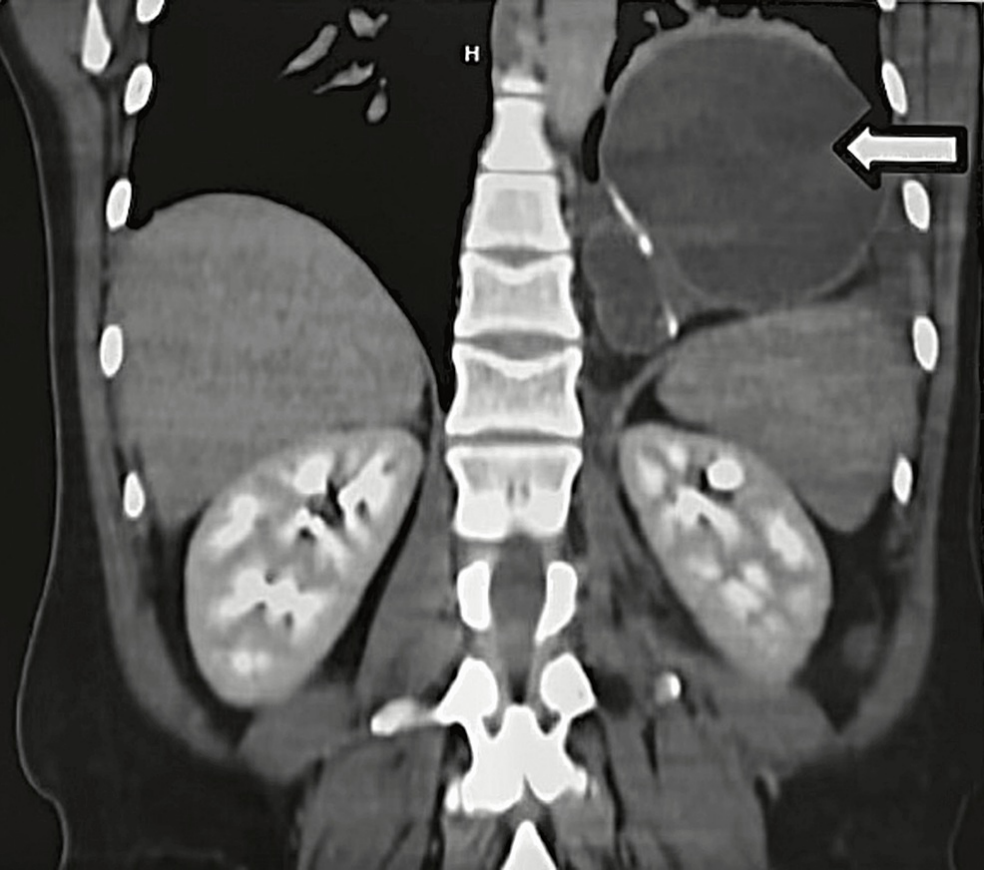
CT scan showing herniated remnant stomach in the left chest (marked with an arrow).
